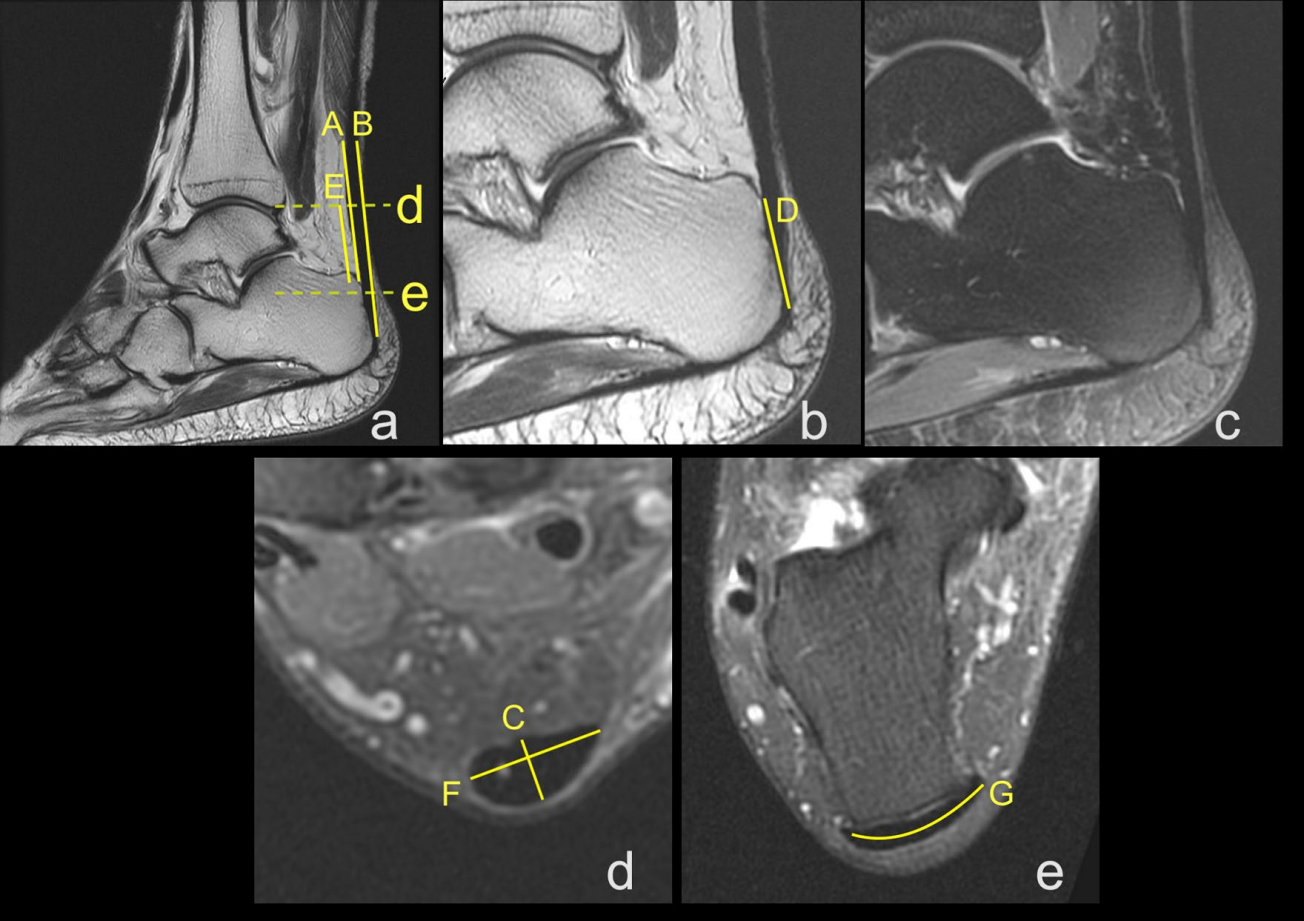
Insertion of the left Achilles tendon. A 61-year-old male who suffered from Achilles pain for eight months, with acute Achilles tendon pain one week before examination. Tendinopathy with partial rupture was suspected. A, B, C, D, F, G—dimensions of the Achilles tendon; definitions in the Material and Methods. (a) and (b) Sagittal T2-weighted FSE (fast spin echo), (c)- sagittal PD-weighted FS (fat suppression), (d) and (e) axial PD-weighted FS.
